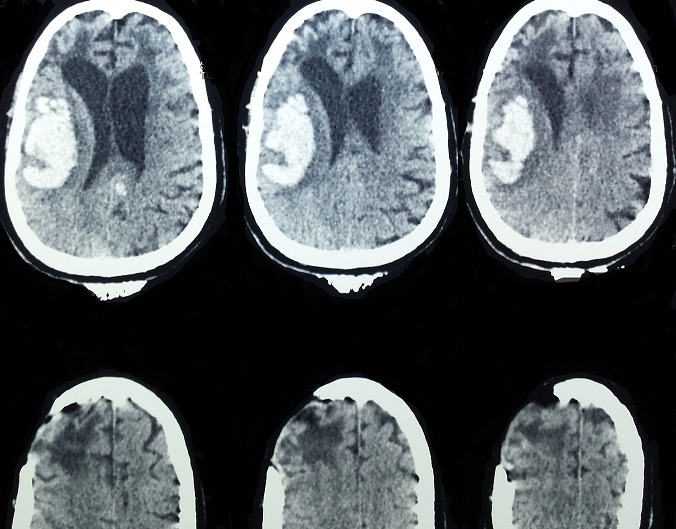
Cranial CT-scan showing an acute right parieto-temporal hematoma, sequelar frontal hypodensity of the old stroke with its cranial flap
